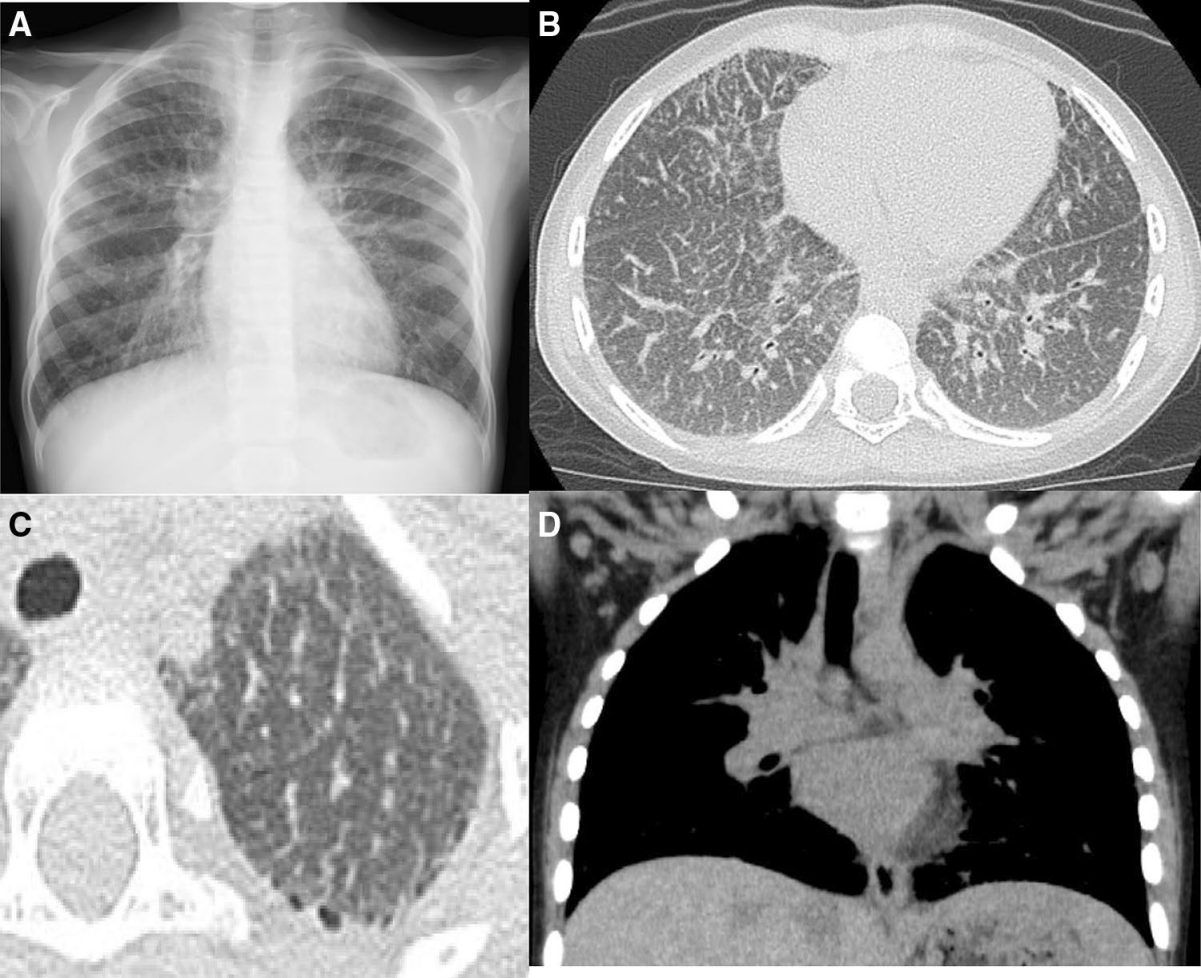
Lung involvement in lysinuric protein intolerance. An 8-year-old boy with postnatal growth failure, osteoporosis noted in early childhood, and aversion to protein rich food. The diagnosis was confirmed on molecular grounds. A CXR showed reticular shadows in both lungs. B,C CT revealed patchy GGOs and thickening of interlobular septa and bronchovascular bundles, reflecting alveolar proteinosis itself and fibrosis that developed over time. Presence of subpleural cysts suggests secondary alveolar growth impairment. D Mediastinal and hilar lymphadenopathy was evident
